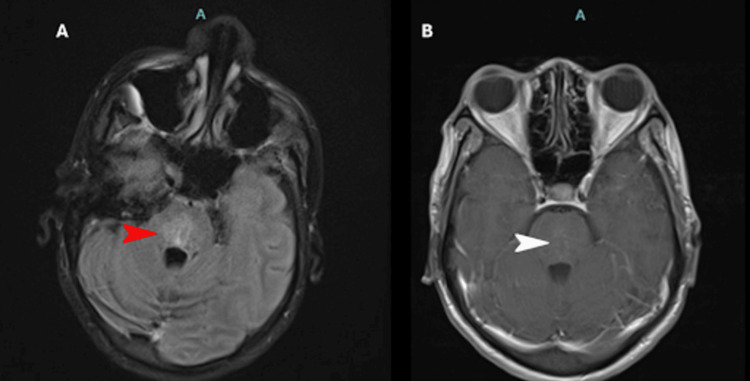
Brain MRI with and without contrast three weeks after admission (A) Decreased pons hyperintensity in axial FLAIR sequence (red arrowhead). (B) Minimal contrast enhancement compared with initial brain MRI (white arrowhead).
